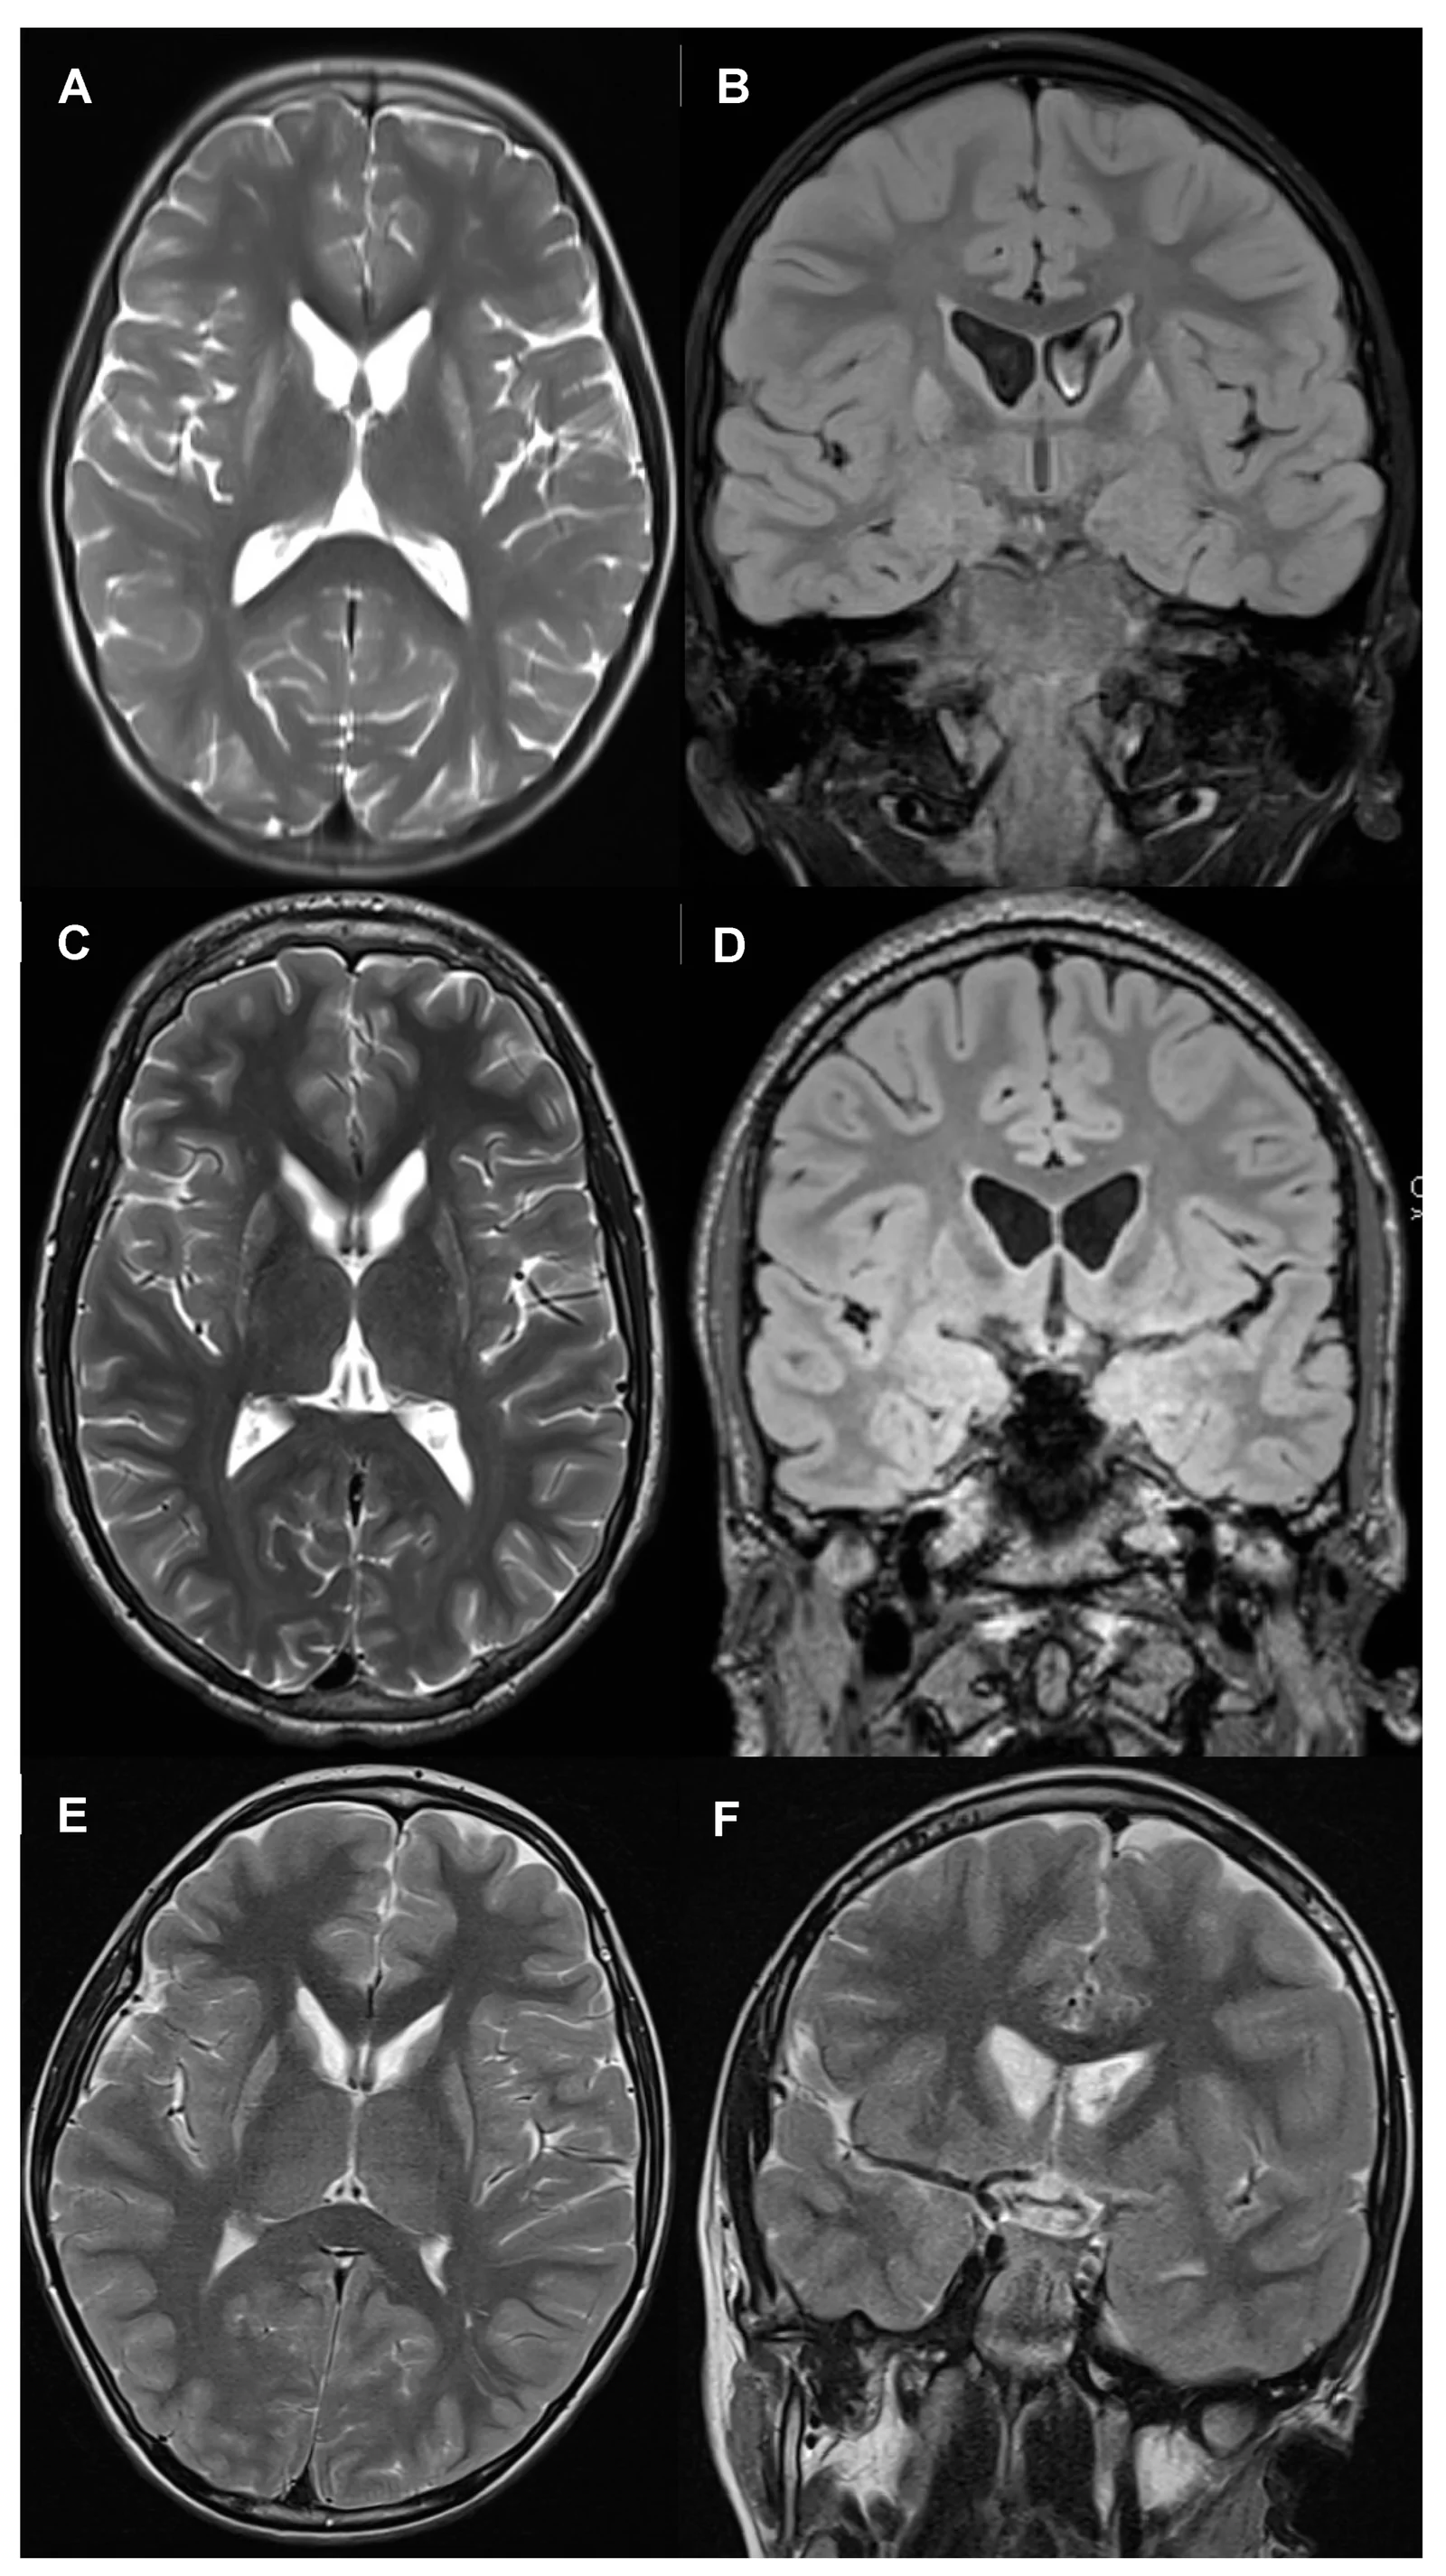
Brain MRI findings in the three patients with juvenile-onset Huntington disease. (A) Axial T2-weighted image showing atrophy of the lentiform nuclei in Patient 1. (B) Coronal fluid-attenuated inversion recovery (FLAIR) image showing atrophy of the caudate heads in Patient 1. (C) Axial T2-weighted image showing atrophy of the lentiform nuclei in Patient 2. (D) Coronal FLAIR image showing severe atrophy of the caudate heads in Patient 2. (E) Axial T2-weighted image showing increased signal intensity in the bilateral putamina and caudate nuclei in Patient 3. (F) Coronal T2-weighted image showing atrophy of the caudate nuclei in Patient 3.
